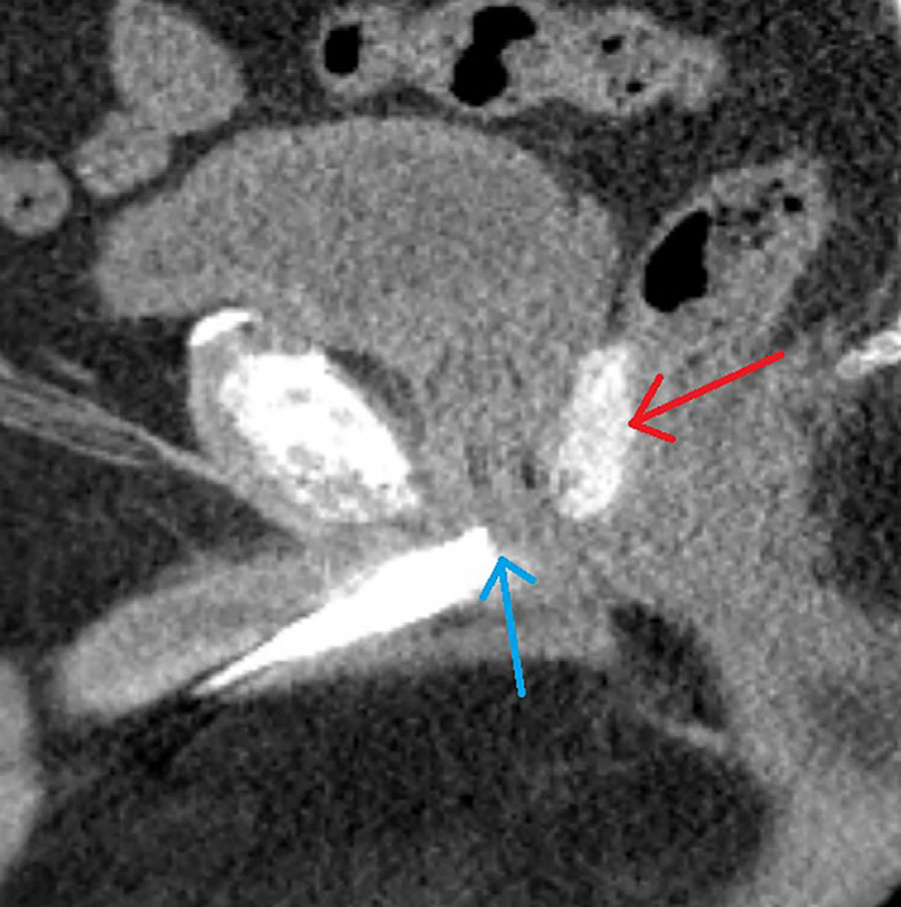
A 69-year-old male with intermediate risk prostate cancer had an implantable cardiac pacemaker which precluded magnetic resonance imaging for treatment planning. Thus he underwent placement of SpaceOAR Vue™ iodinated rectal spacer and a urethrogram CT image was obtained for treatment planning: Treatment planning sagittal computed tomography urethrogram images demonstrate the radiopaque spacer between the prostate and rectum (red arrow), as well as the beak of the urethrogram (blue arrow).
